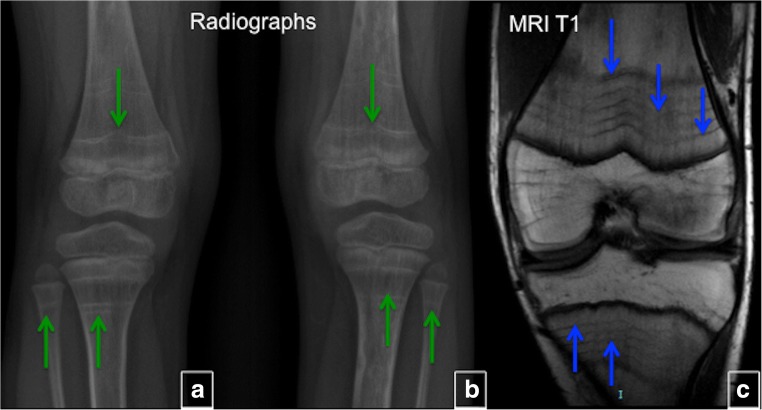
Bisphosphonate-induced lines. Administration of bisphosphonates results in the development of the parallel sclerotic metaphysial bands, which can be seen on radiographs (*green arrows*) and T1-weighted MRI (*blue arrows*)
